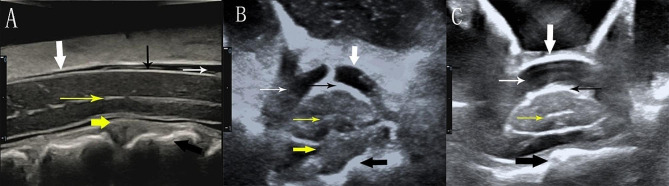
Intraoperative sagittal and cross-sectional sonograms of the SC. (A) Sagittal image. (B) Transverse image of the CA. (C) Transverse image of the NCA. The distance from 0 to 1 on the scale represents 1 centimeter. The spinal dura mater (bold white arrow), pia mater (thin black arrow), subarachnoid space (thin white arrow), SCCEC (thin yellow arrow), anterior compression element (bold yellow arrow), and posterior margin of the cervical vertebra (bold black arrow) could all be clearly visualized. Area_compressed_ was measured on transverse images in the CA (B); Area_free_ was measured on transverse images in the NCA (C)
